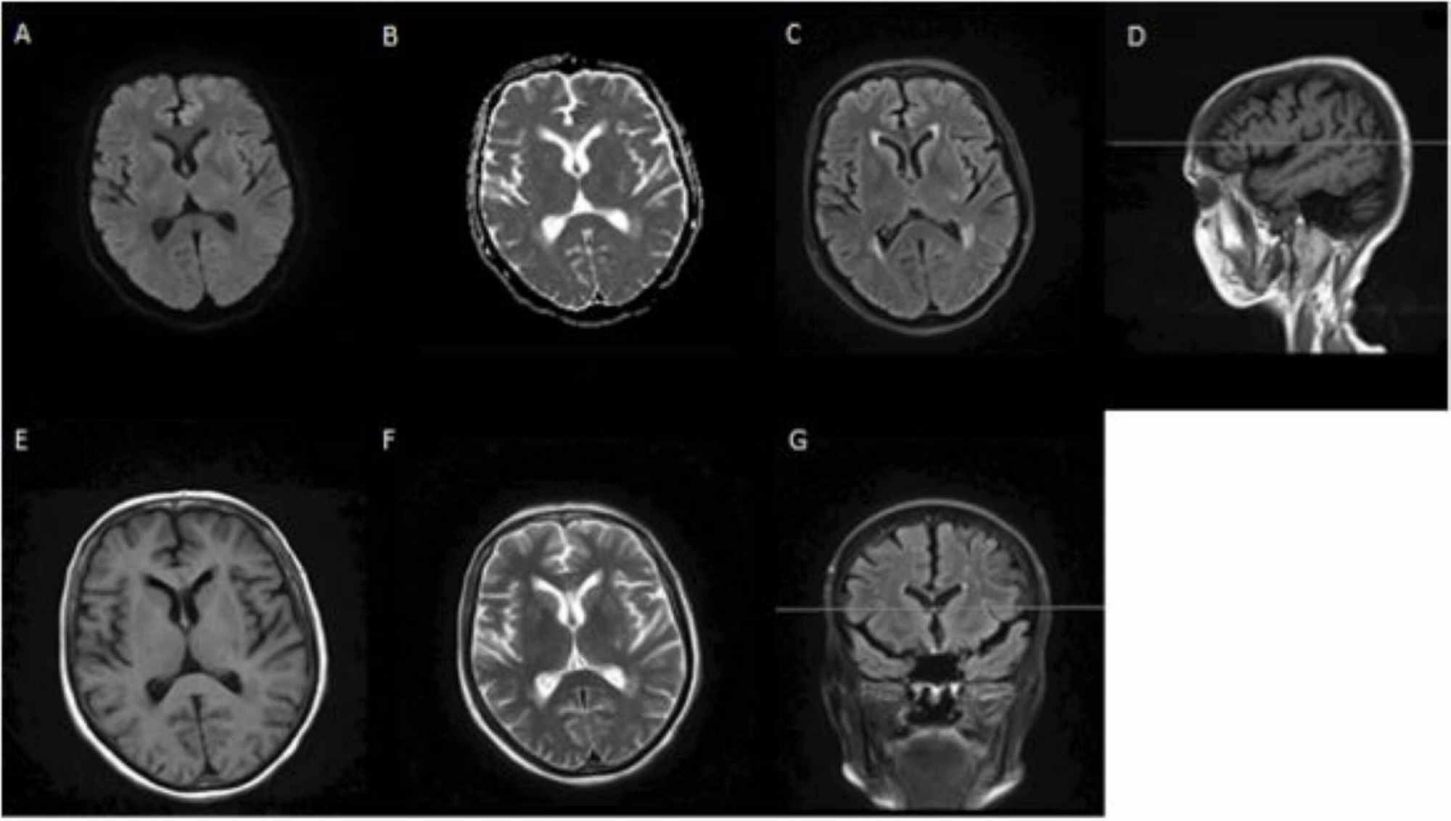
MRI of the brain without intravenous contrast A: axial DWI; B: axial ADC; C: axial T2 FLAIR; D: sagittal T1 FLAIR; E: axial T1 FLAIR; F: axial T2; G: coronal T2 FLAIR Mild age-appropriate generalized cerebral atrophy. No acute infarction or foci restricted diffusion are identified in DWI and ADC maps. Nonspecific and chronic supratentorial white matter changes are seen MRI: magnetic resonance imaging; DWI: diffusion-weighted imaging; ADC: apparent diffusion coefficient; FLAIR: fluid-attenuated-inversion-recovery
